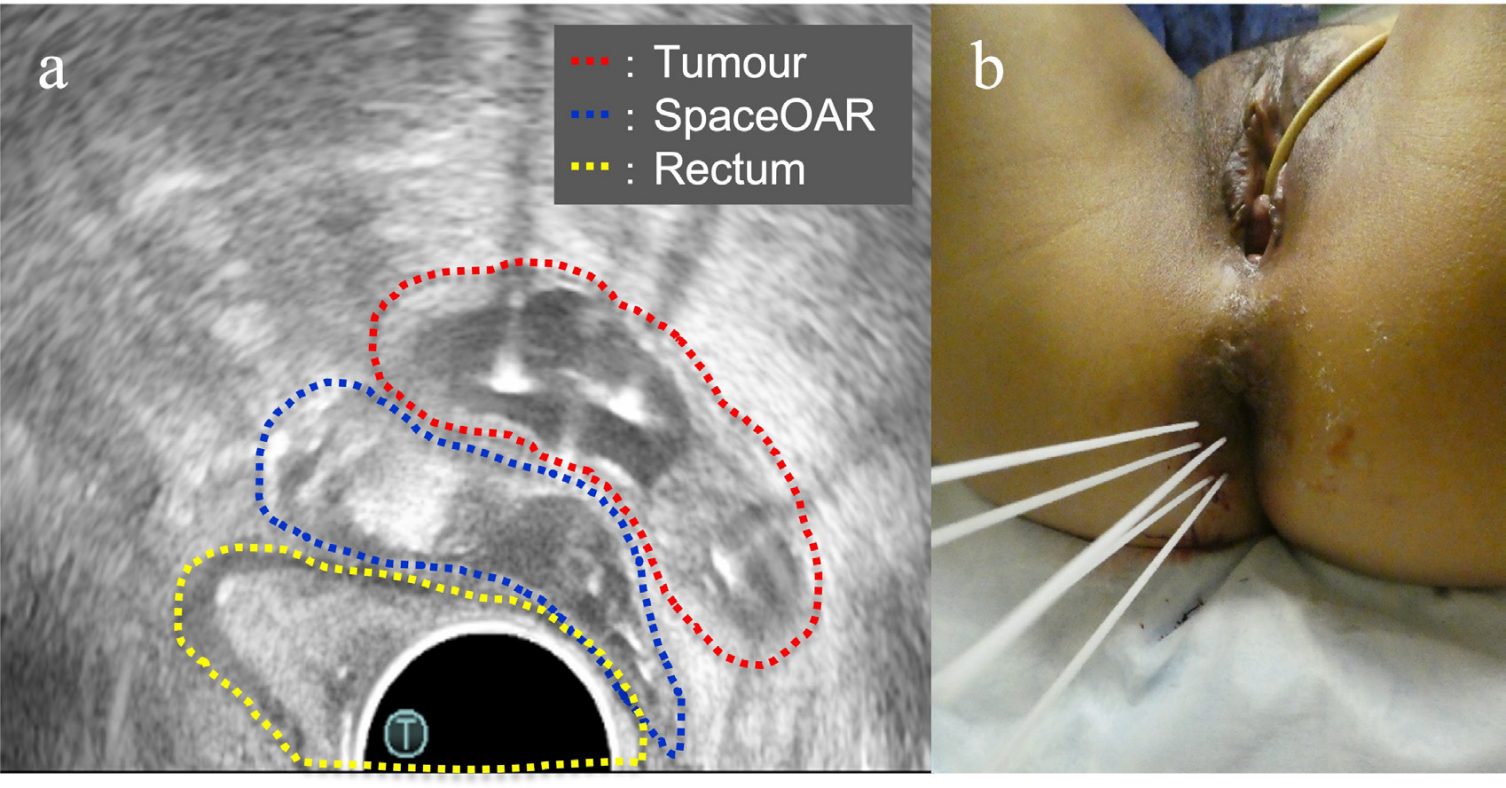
(a) Axial view of the transrectal ultrasound after implantation of the SpaceOAR hydrogel spacer and interstitial needles. This shows that the SpaceOAR is placed in an optimal position between the tumour and rectum; (b) Image of the interstitial brachytherapy.
